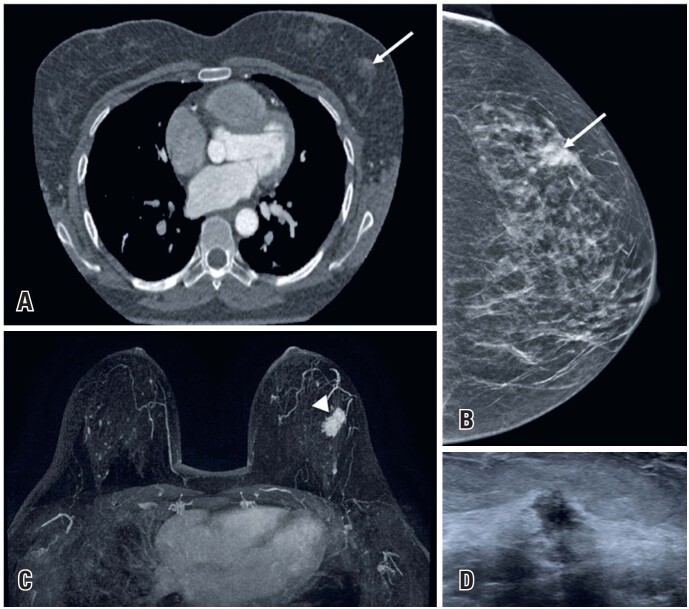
A 49-year-old female underwent a routine coronary CTA. (A) No coronary abnormalities were observed. However, an asymmetric density was identified in the left breast (arrow). (B) Mammography revealed an irregular spiculated mass, classified as BI-RADS 4 (arrow). (C) The lesion was evident on the MRI T1-weighted gadolinium-enhanced MIP sequence (arrowhead). (D) Ultrasound demonstrated a hypoechoic, irregular, angulated mass. Subsequent biopsy confirmed the diagnosis of invasive ductal carcinoma
